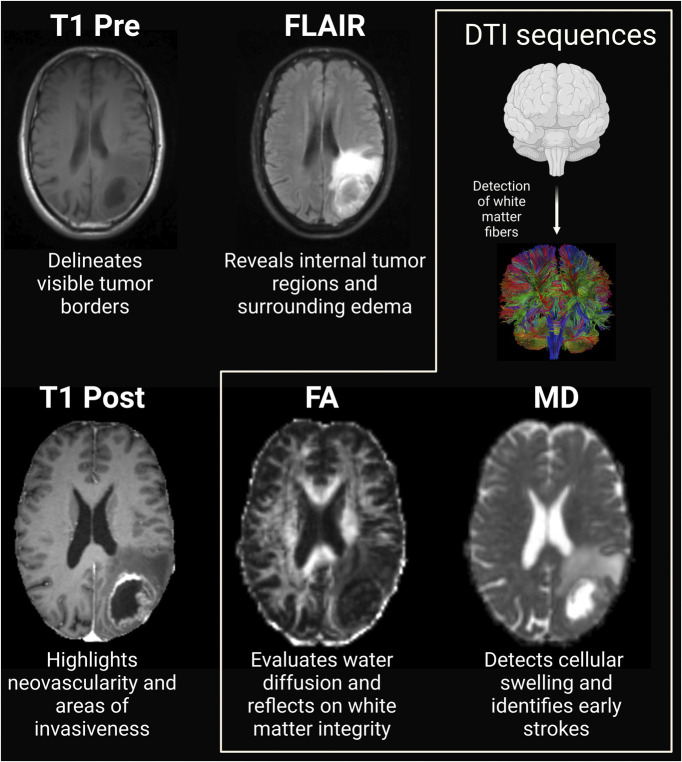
MRI and DTI sequences for glioblastoma characterization. T1 Pre delineates visible tumor borders, FLAIR and T2 highlight internal tumor regions and edema. Contrast enhancement on MRI indicates neovascularity and infiltration. DTI assesses tissue microstructure through water diffusion, evaluating white matter tract integrity using MD and FA. MD detects cellular swelling, aiding early stroke detection, while FA measures water diffusion orientation, reflecting white matter integrity. DTI, diffusion tensor imaging; FA, fractional anisotropy; FLAIR, fluid-attenuated inversion recovery; MD, mean diffusivity.
